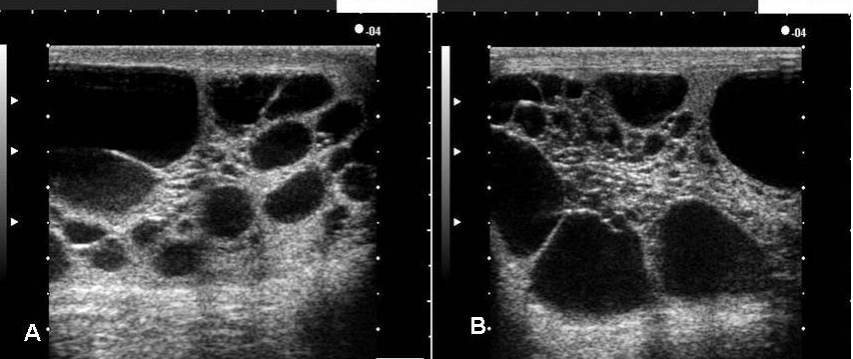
Ultrasonography showed that both testes had undergone overall degeneration to multilocular cysts, and there was overall enlargement of the testes.
